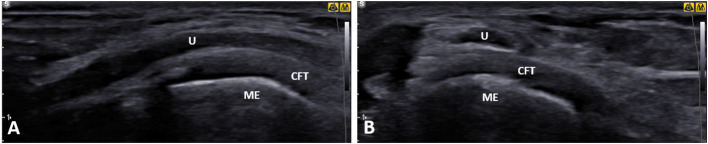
Ultrasound (*US*) imaging of the ulnar nerve (*U*) at the level of the medial epicondyle (*ME*) in a patient with symptomatic ulnar nerve dislocation. Both longitudinal (A) and short-axis (B) views clearly demonstrate a close contact between the ulnar nerve and common flexor tendon origin. U, ulnar nerve; CFT, common flexor tendon; ME, medial epicondyle.
